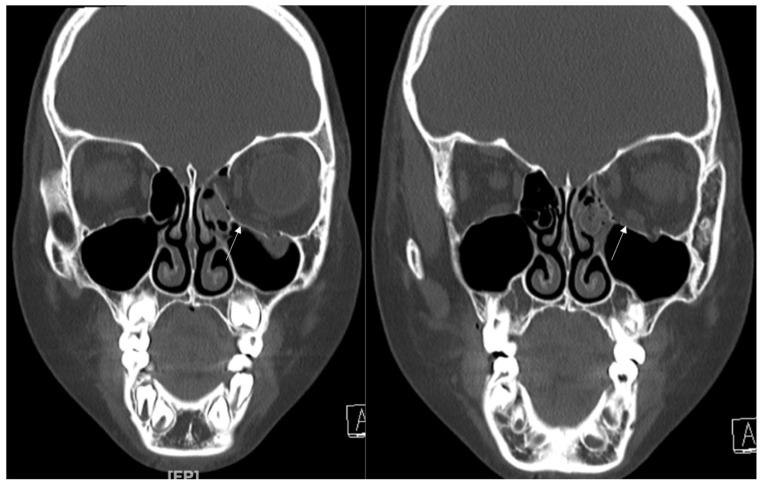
Case 1. Preoperative facial CT scan (coronal view) of the 8-year-old male patient with left inferomedial orbital wall fracture. The fracture sites were designated with white arrows.
